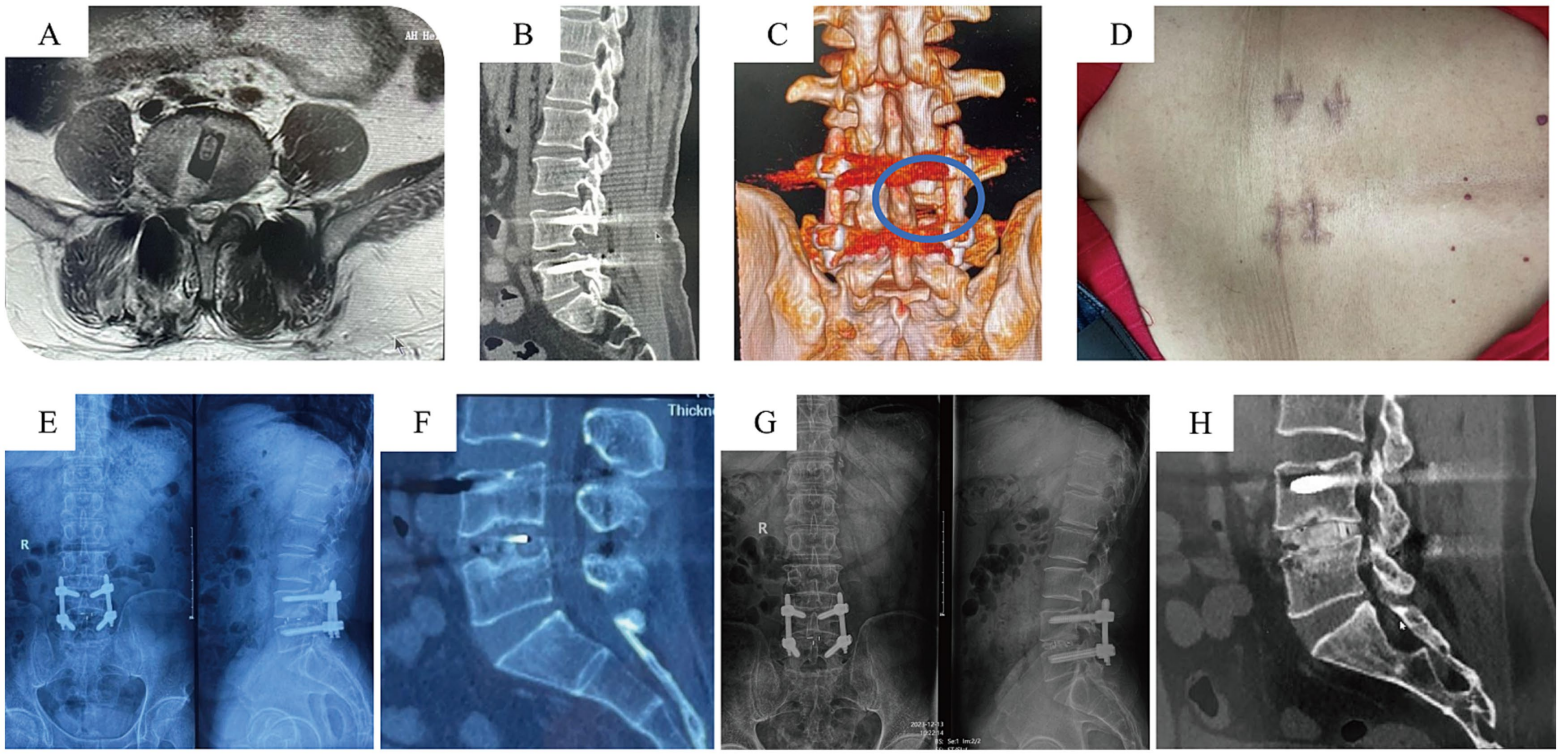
Postoperative follow-up data for UBE-TLIF Case. (A–C) Postoperative MRI indicating adequate decompression; CT showing the osteotomy surfaces of the superior and inferior articular processes. (D) Gross photo of the surgical wound. (E,F) X-ray and CT at 6 months postoperatively. (G,H) X-ray and CT at 2 years postoperatively indicating satisfactory fusion.
